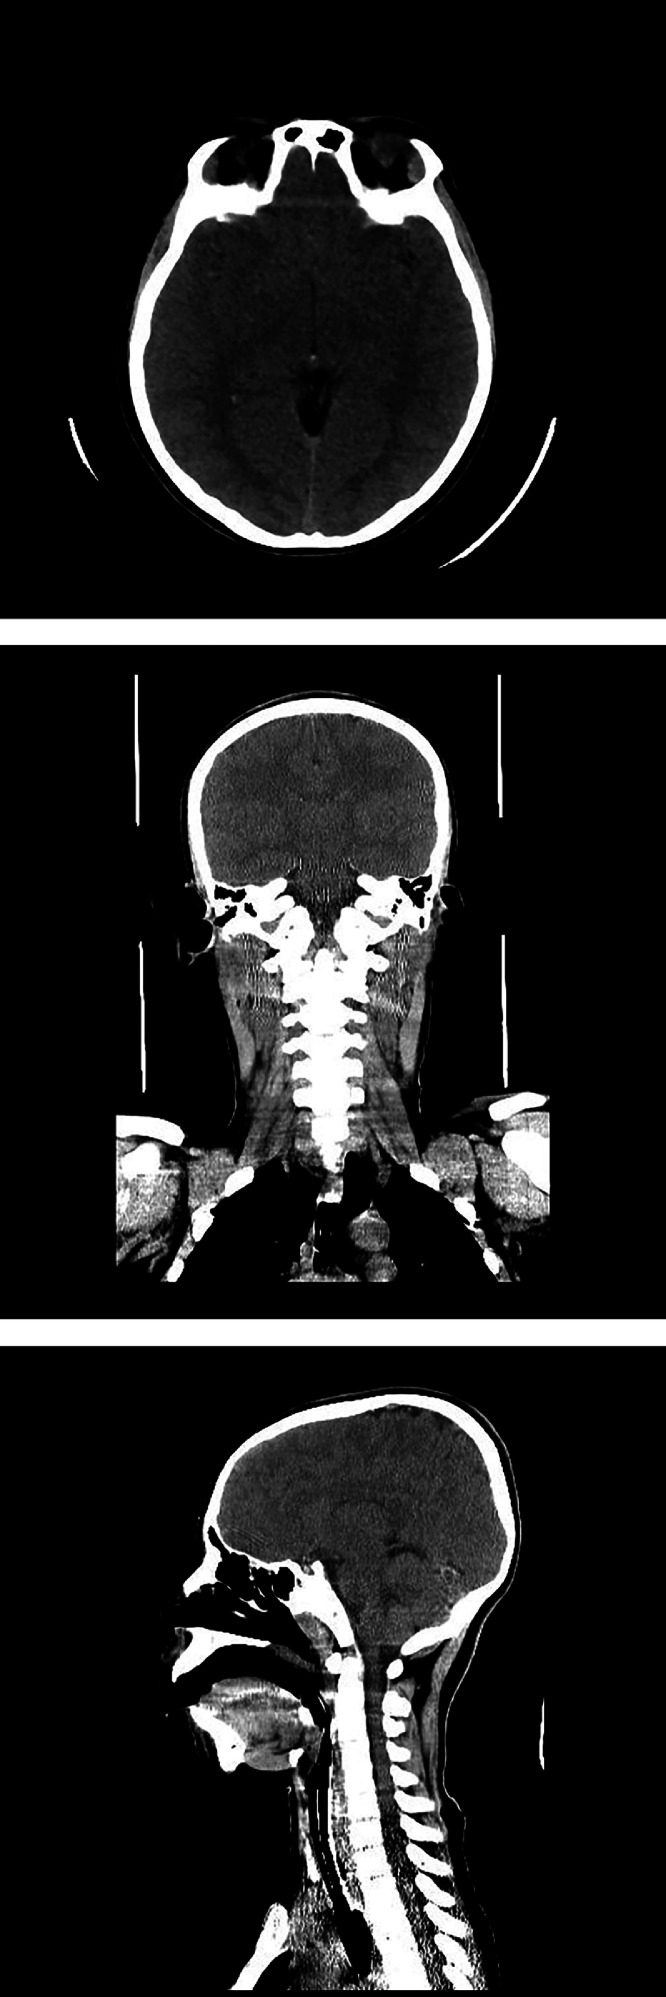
Noncontrast brain CT of the patient.
